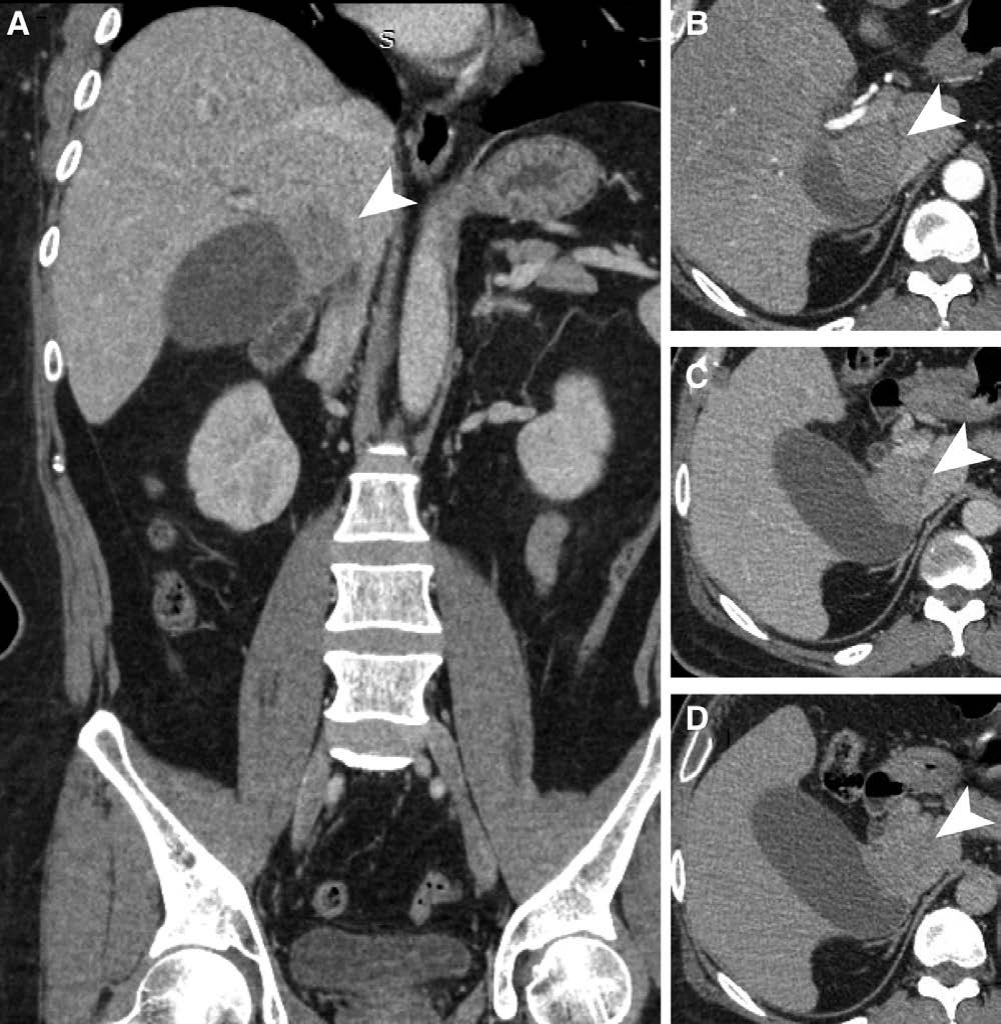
Multiphase contrast-enhanced CT scans. (A) A solid mass in the cystic duct on the coronal plane; (B–D) cross-sectional contrast-enhanced CT shows that the mass was unevenly enhanced, with hypodense shadows that persisted until the delayed phase: (B) arterial phase, (C) venous phase, and (D) delayed phase. Arrows indicate the mass.
